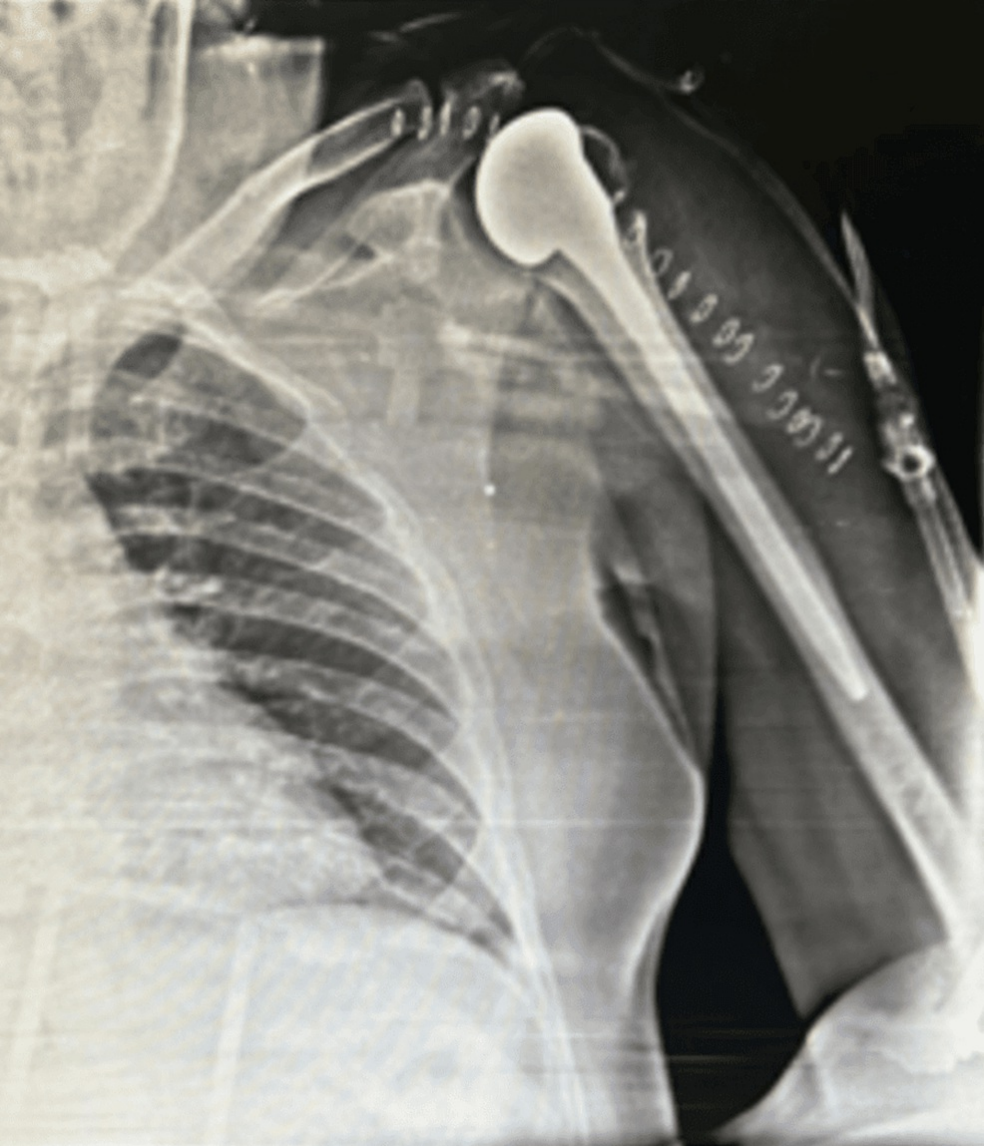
Post-operative X-ray of the left shoulder joint
